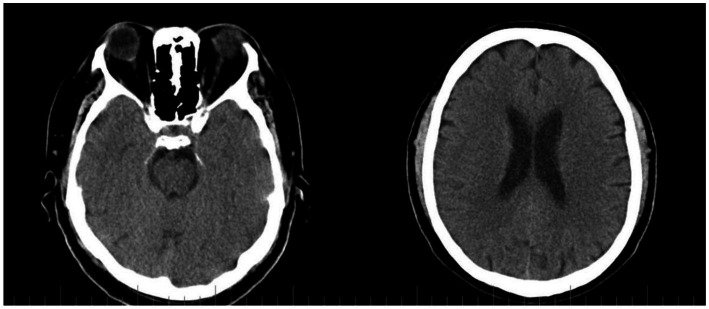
CT scan with no pathological findings.
